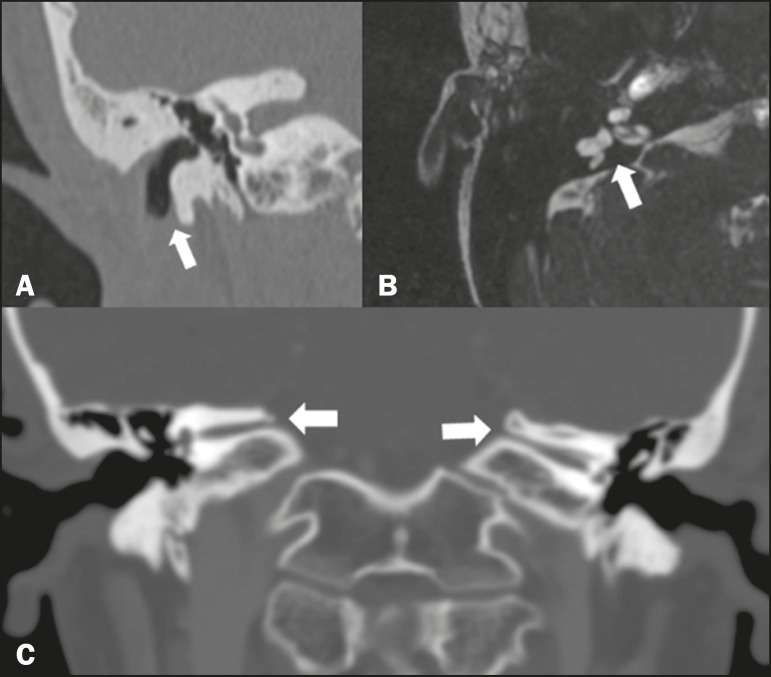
Congenital malformations. A: Coronal CT scan, with bone
